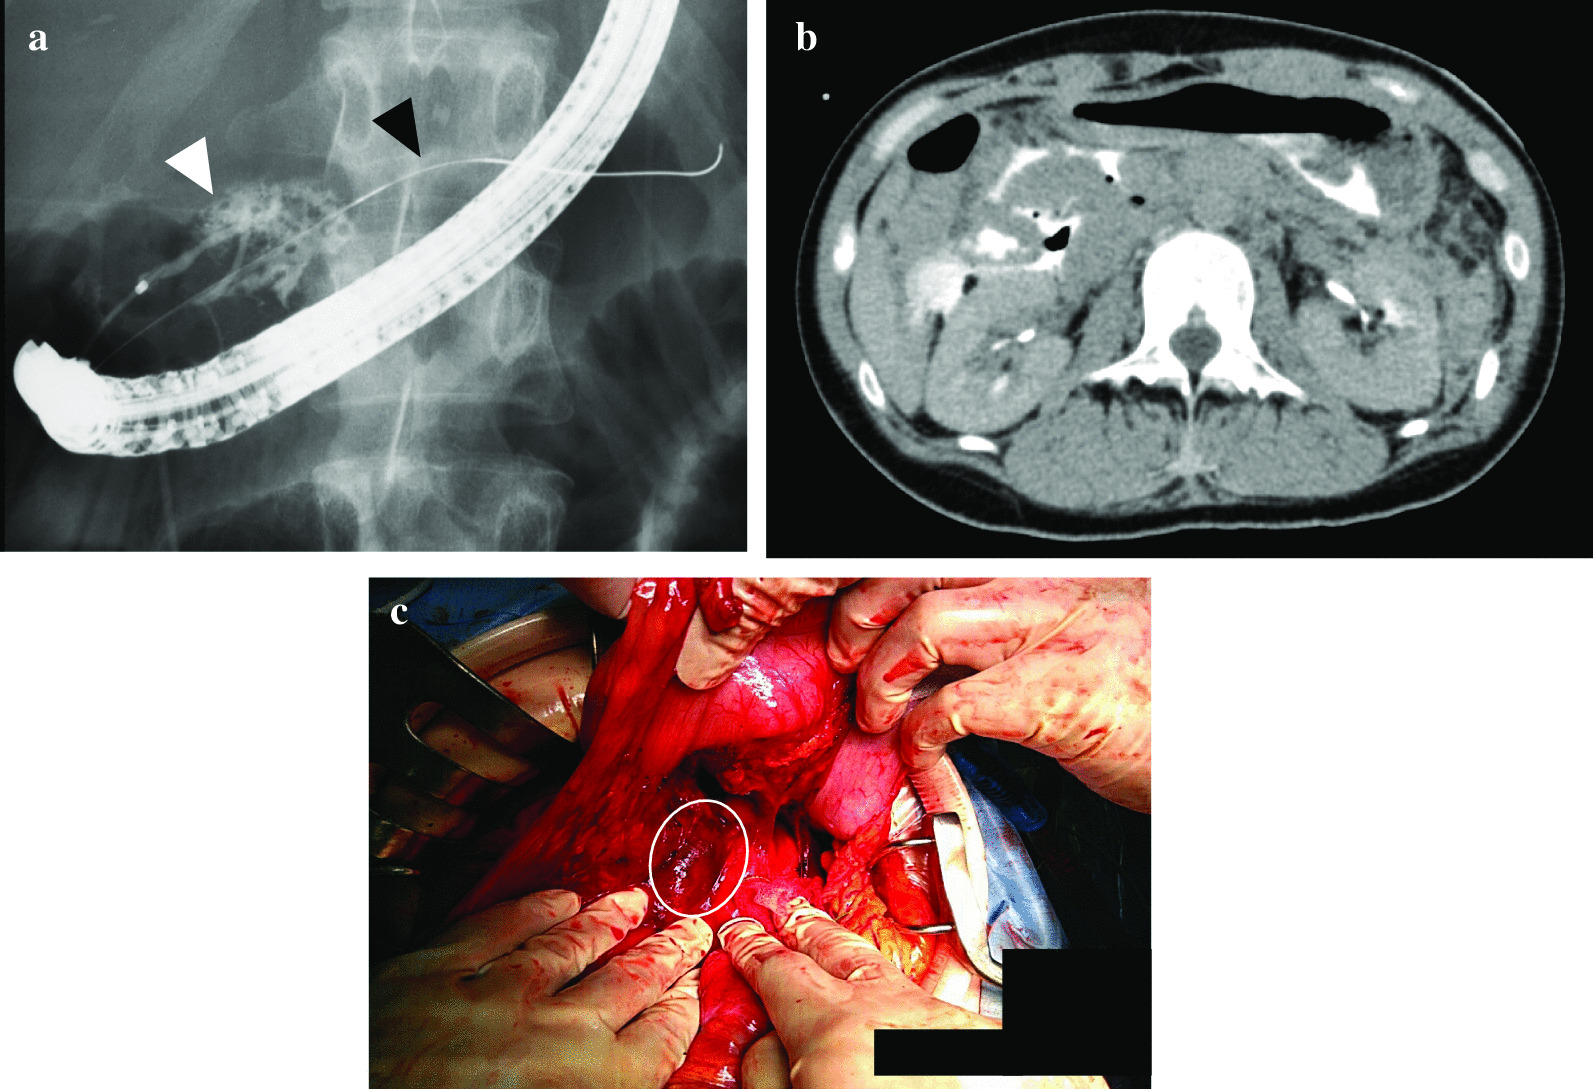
(a) Endoscopic retrograde pancreatography (ERP) findings, (b) CT findings after ERP, and (c) the findings during damage control surgery and views of the surgical site. Endoscopic retrograde pancreatography (ERP) findings in semi-prone position: Major leakage was found from the main pancreatic duct when contrast medium was injected from the ampulla of Vater (white arrowhead). Although the guidewire (black arrowhead) was able to be placed in the distal pancreatic duct, EPST and ENPD tube could not be cannulated to the distal duct over the injured sight. (b) Abdominal CT findings following ERP: abdominal CT revealing major leakage of contrast media and free air intraperitoneal and retroperitoneal space around the pancreas following ERP. (c) The findings during damage control surgery and views of the surgical site: a laceration with a diameter of approximately 2.5 cm and hemorrhage found on the cranial side of the pancreatic head by Kocher’s maneuver and entering omental bursa
